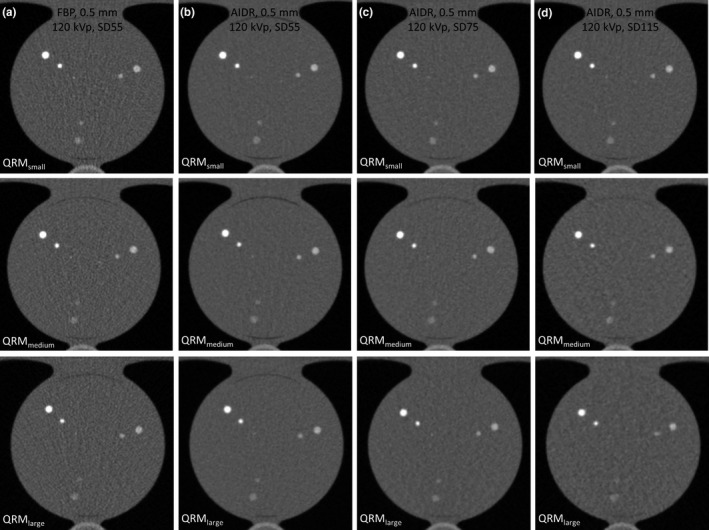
CT images acquired with four different acquisition/reconstruction combinations for QRM_small_ (top row), QRM_medium_ (mid row), and QRM_large_ (bottom row): (a) FBP, 0.5 mm, 120 kVp, SD = 55; (b) AIDR, 0.5 mm, 120 kVp, SD = 55; (c) AIDR, 0.5 mm, 120 kVp, SD = 75; and (d) AIDR, 0.5 mm, 120 kVp, SD = 115
